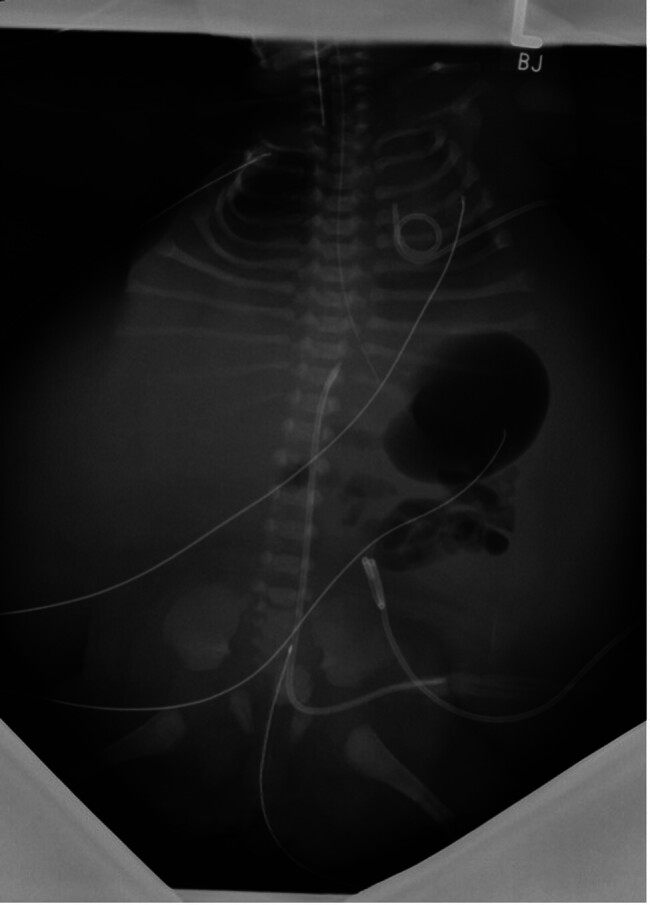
X-ray examination of thorax and abdomen of the infant in anterior–posterior projection on the first day of life. The photograph showed a massive abdominal distension due to urinary ascites, a bell-shaped thorax, an air-filled stomach, as well as proximal intestinal loops.
